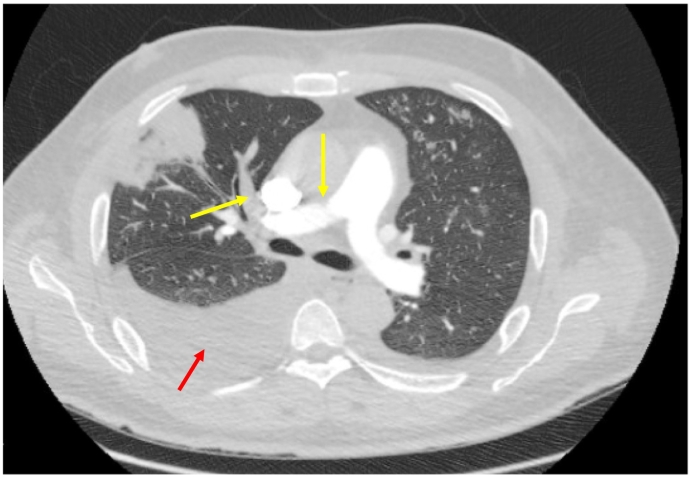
Computed tomography angiography of the chest showing pulmonary embolism with yellow arrows pointing to the pulmonary embolism in the right pulmonary artery and the red arrow pointing to the right pleural effusion.
